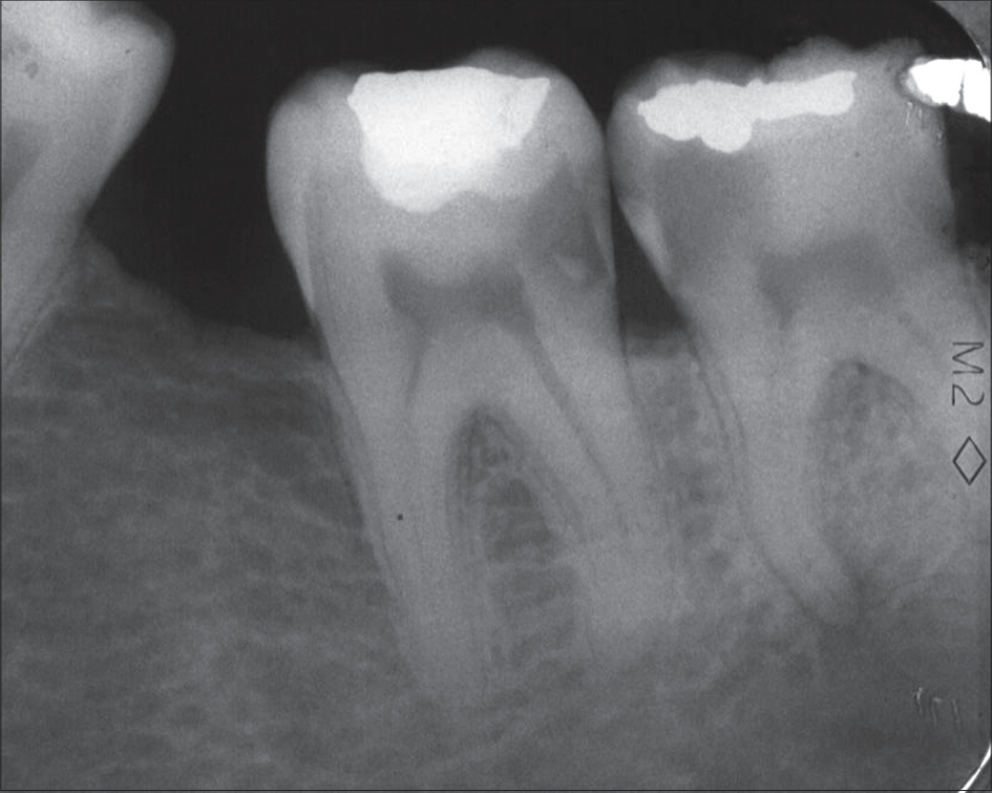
Preoperative radiograph of the second and third lower left molars shows deep interproximal carious lesions. The patient's chief complaint was severe lingering pain with sensitivity to percussion in both involved teeth. A periradicular lesion in the mesial root of the third molar is clear
